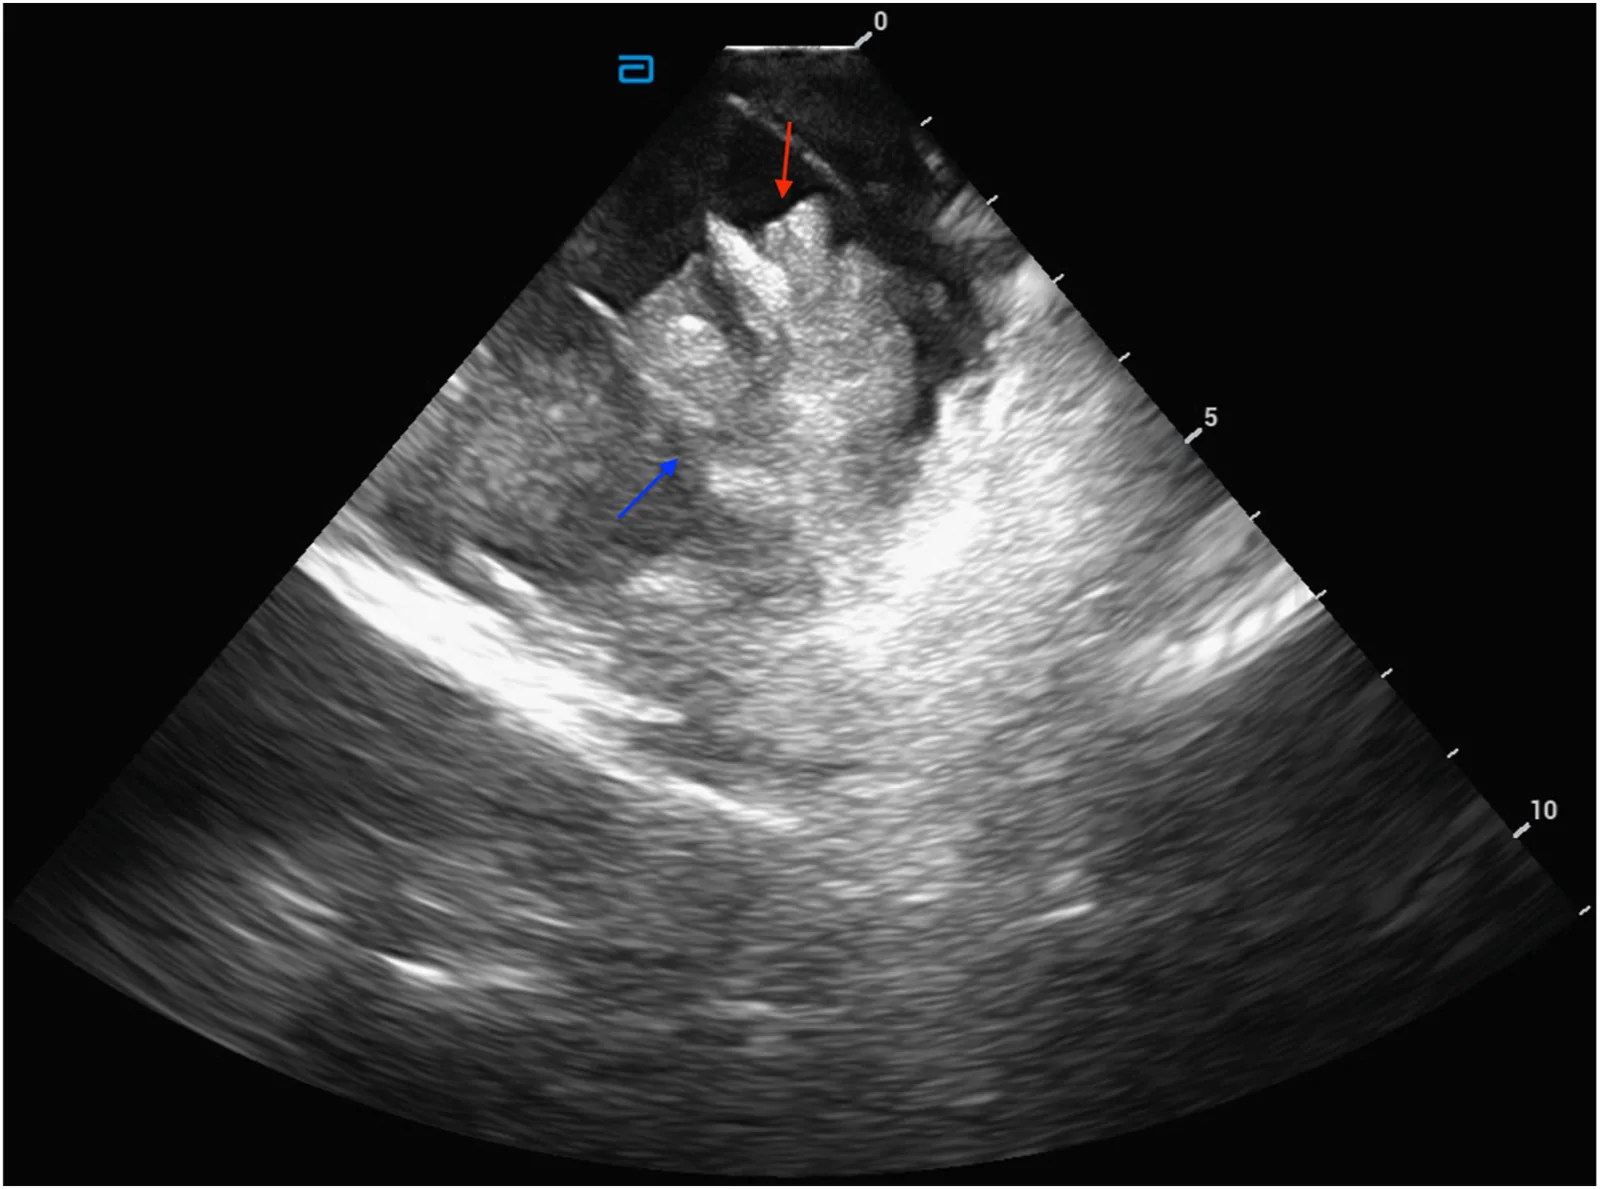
Intracardiac echocardiogram of the right ventricle showing the leadless pacemaker (*blue arrow*) and associated infective vegetation (*red arrow*), with probable involvement of the surrounding papillary muscle.
